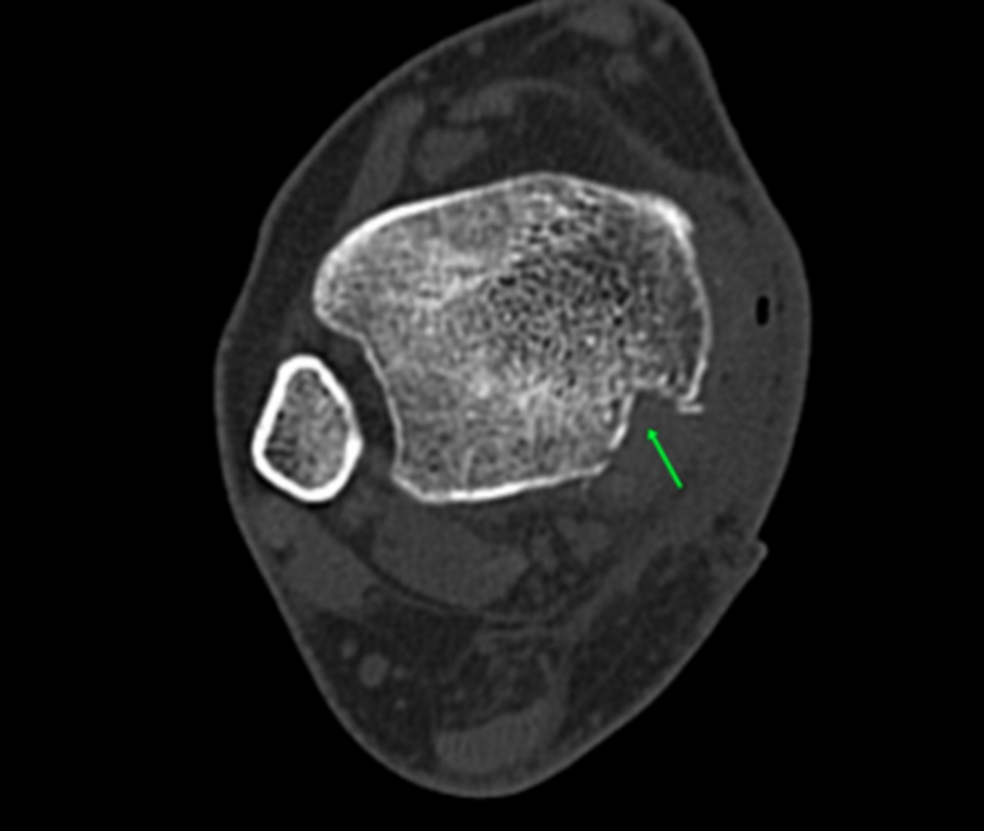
CT scan (axial view) of the right ankle depicting the deepened retromalleolar groove postoperatively (green arrow). CT: Computed Tomography
